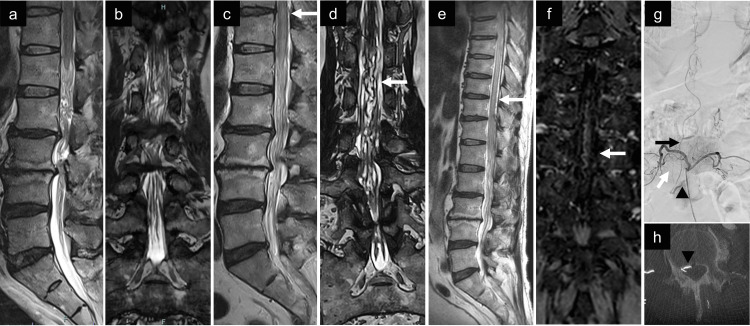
Preoperative radiological findings. (a) Initial sagittal T2-weighted MRI showing lumbar spinal canal stenosis at the L2/L3 and L3/L4 levels, with faint intramedullary hyperintensity. (b) Initial coronal FIESTA MRI showing lumbar spinal canal stenosis at the L2/L3 and L3/L4 levels, without abnormal vessels. (c) Sagittal T2-weighted MRI after laminectomy showing resolution of the spinal canal stenosis, but a slight flow void (white arrow) was observed dorsally to the conus medullaris. Hyperintense lesions in the conus medullaris were also observed compared to the initial image. (d) Coronal FIESTA MRI after showing lumbar spinal canal stenosis at the L2/L3 and L3/L4 levels without abnormal vessels (white arrow). (e) The hyperintense lesion was enlarged longitudinally to the mid-thoracic level. Multiple dorsal flow voids (white arrow) were concurrently observed. (f) Coronal contrast-enhanced MRI demonstrating an abnormal vessel (white arrow) running longitudinally in the thoracic spinal cord. (g) Digital subtraction angiography of the right L2 segmental artery, revealing an epidural arteriovenous fistula fed by the dorsal somatic branch (white arrow). The fistula (black arrowhead) drained into the perimedullary vein (black arrow). (h) The shunt pouch (black arrowhead) was located in the epidural space on the axial image of the rotational three-dimensional angiography. MRI: magnetic resonance image; FIESTA: fast imaging employing steady-state acquisition
